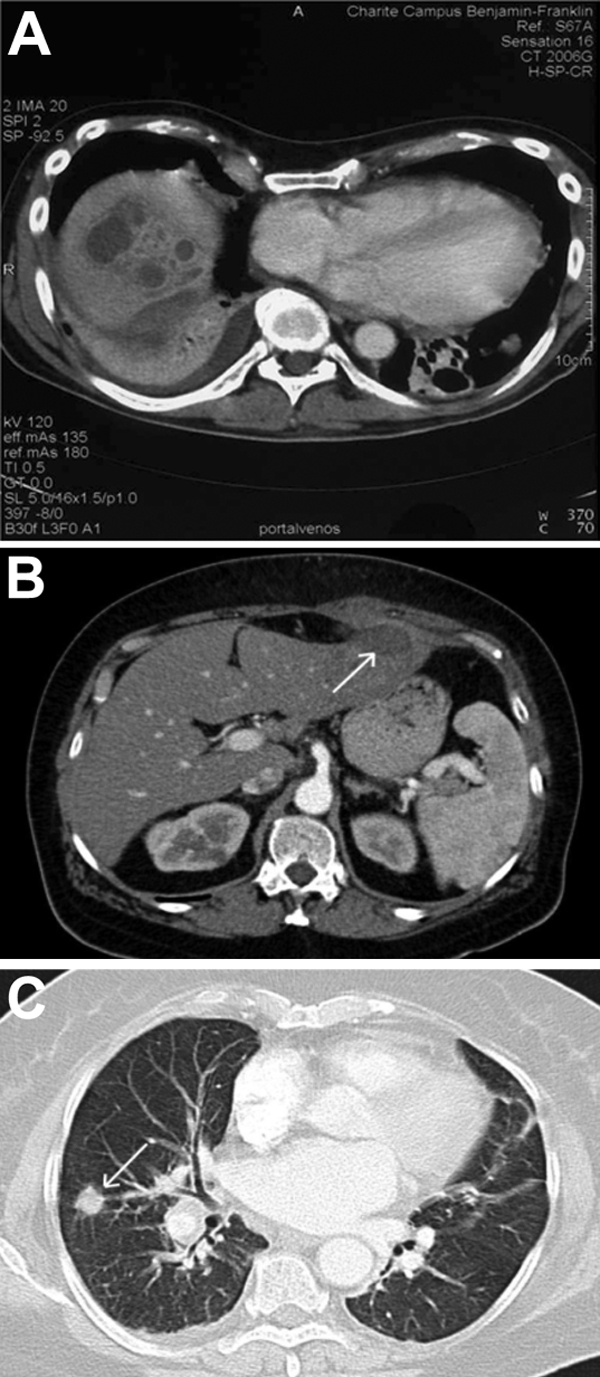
Figure. Pretreatment computed tomography images of patients with *Klebsiella pneumoniae* liver abscesses. A) Multilobular abscess in segment 8 of the liver and pleural effusion in 48-year-old white man (patient 1). B) Liver abscess (arrow) in 71-year-old white woman with type-2 diabetes (patient 2). C) Lung lesion (arrow) in patient 2.
